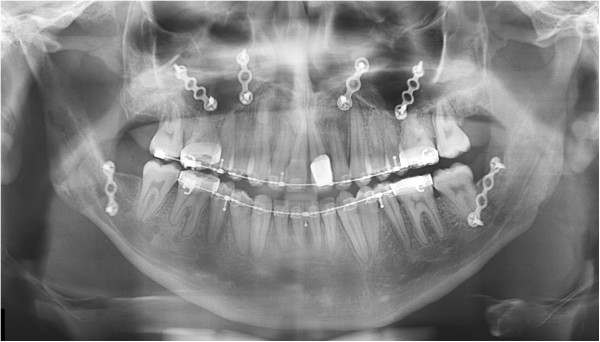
Panoramic radiograph after two-jaw surgery.
